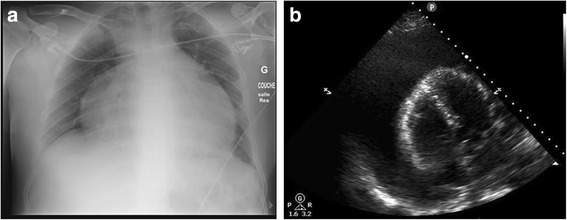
a Chest X-ray showing enlarged cardiac silhouette; b POCUS picture revealing a very large pericardial effusion
